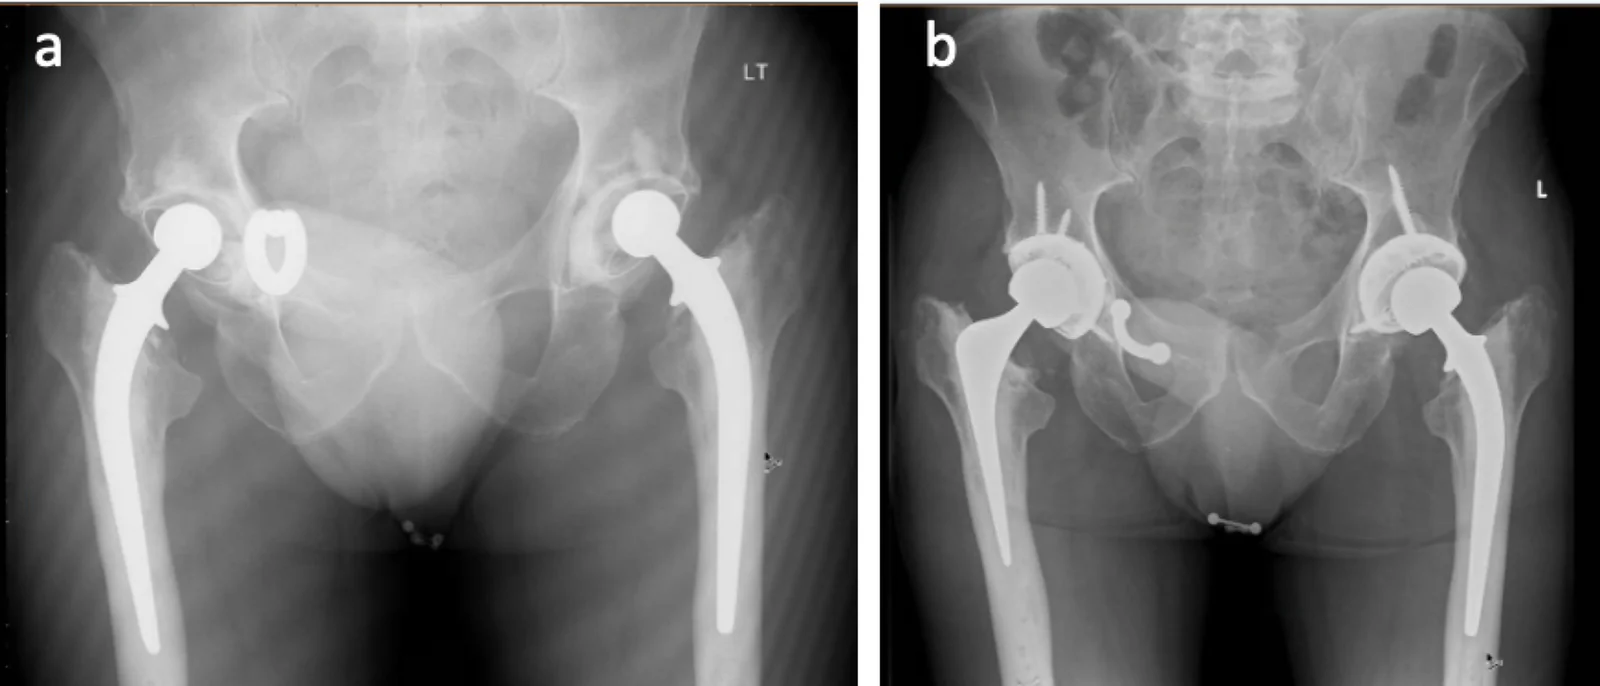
Anteroposterior radiographs of a right total hip arthroplasty undergoing a cement-in-cement femoral revision. a Preoperative view demonstrating a cemented femoral component within a structurally sound, well-integrated cement mantle. b Postoperative view following stem extraction and successful cement-in-cement reimplantation of a new femoral component within the retained cement matrix
